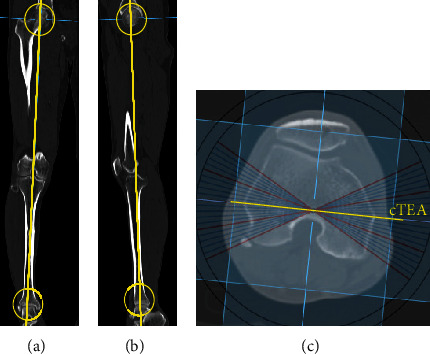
Creation of digitally reconstructed radiographs: reconstruction of 2D images from 3D CT images. The center of the femoral head to the center of the talus surface was set as the vertical axis in the coronal plane (a) and sagittal plane (b), and the clinical transepicondylar line was set as the horizontal axis (c).
